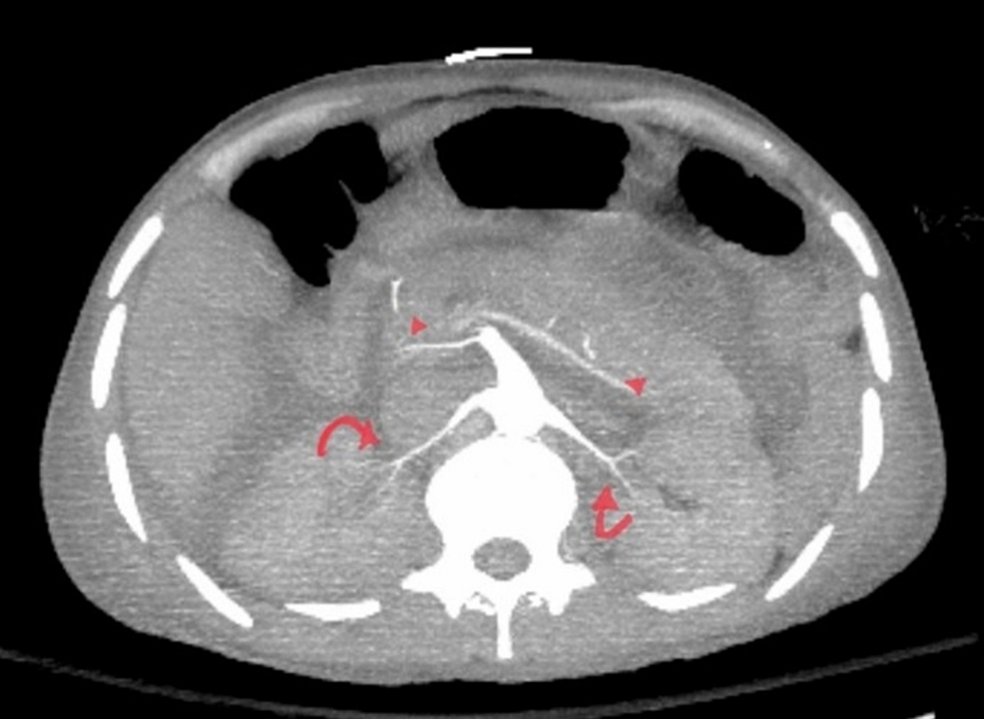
Computed tomography of abdomen/pelvis with angiogram showing tapering of celiac terminals (arrowheads) with significant narrowing of bilateral renal arteries (curled arrows)
